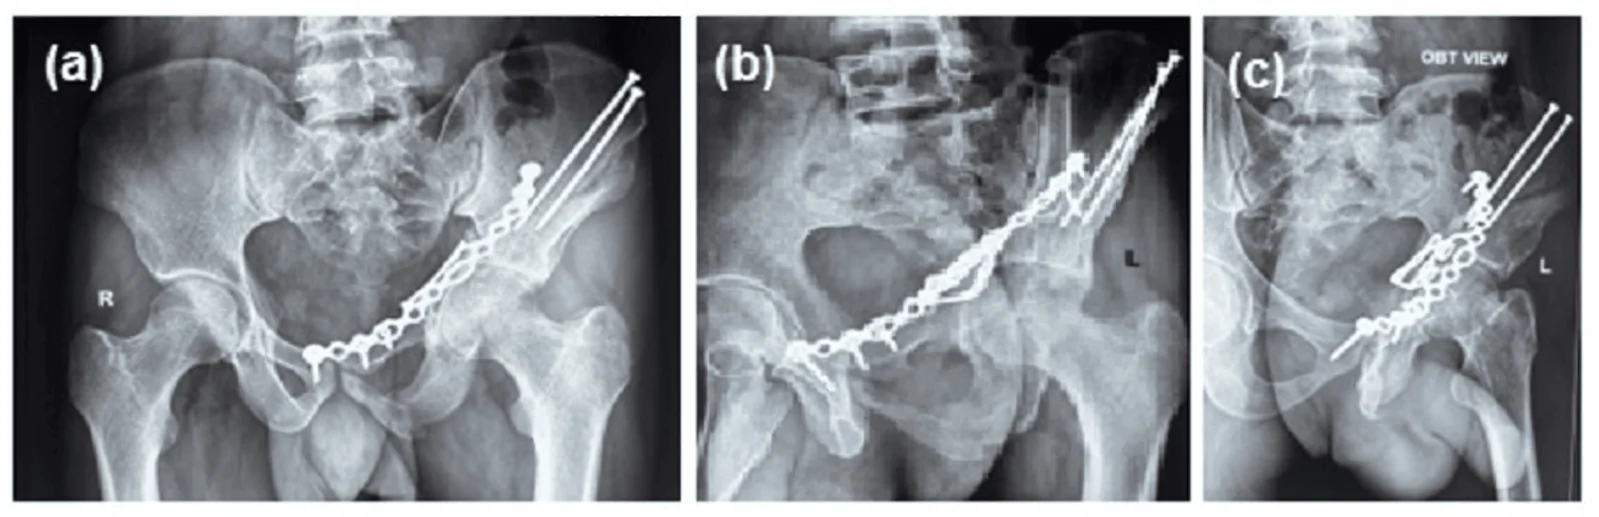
Case 1: Immediate postoperative assessment. (a) Immediate postoperative anteroposterior pelvic radiograph. (b) Immediate postoperative iliac oblique (Judet) radiograph. (c) Immediate postoperative obturator oblique (Judet) radiograph.
